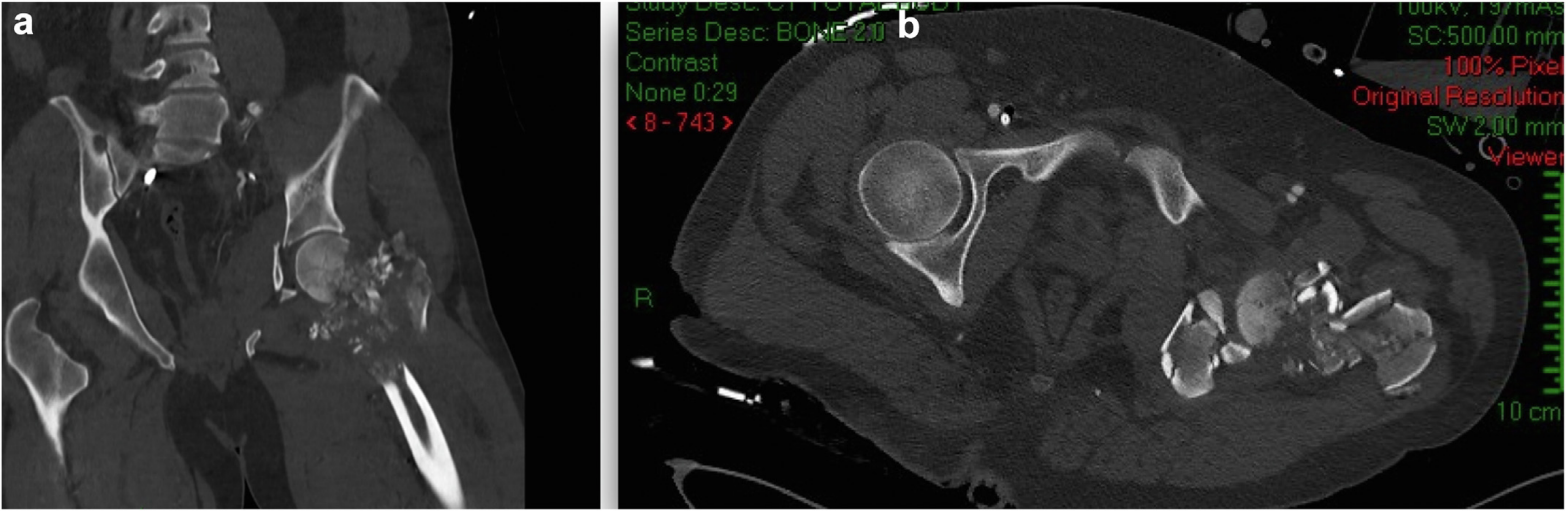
(a) Coronal and (b) axial CT scan cuts of the left femoral head and acetabulum upon admission. Severe periarticular comminution of the left proximal femur and left pelvic part is noted. CT, computed tomography.
